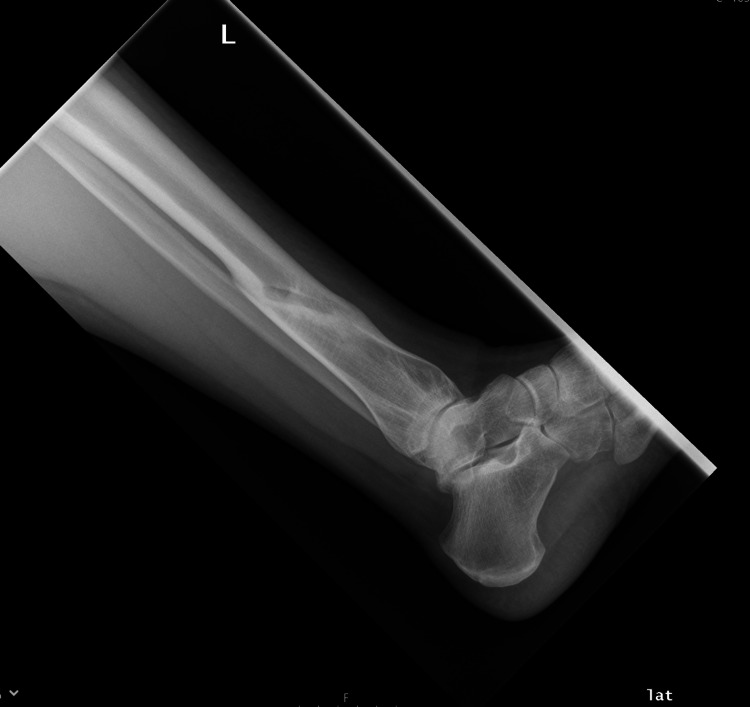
Union status at the completion of 6 months of LIPUS therapy (Lateral view) LIPUS - Low-Intensity Pulsed Ultrasound
